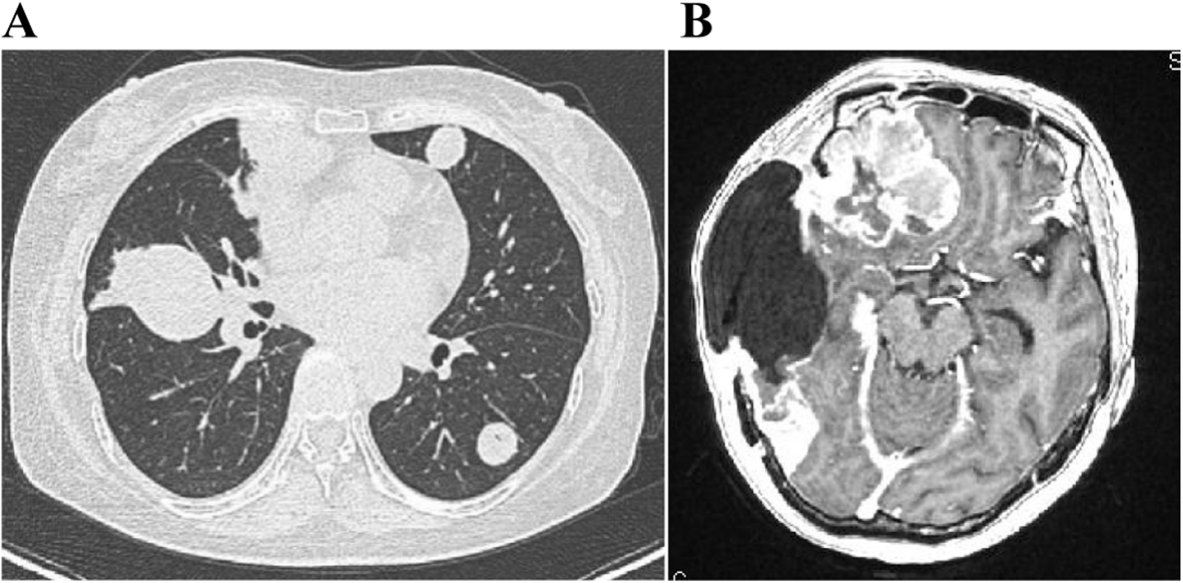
One month after the second craniotomy and puncture biopsy of the lung lesions, A re-examination of the chest radiograph showed enlarged lung metastases. B Intracranial right frontal lobe and occipital lobe lesions grew rapidly and appeared extensive meningeal metastasis
